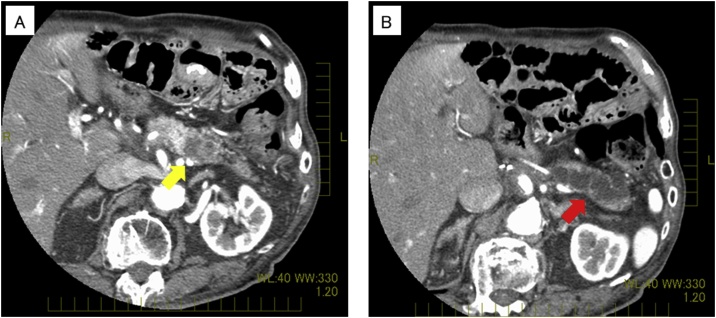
Preoperative computed tomography (CT). (a) Yellow arrow indicates poorly enhanced tumor of the pancreatic body. (b) Red arrow indicates the dilated main pancreatic duct.
